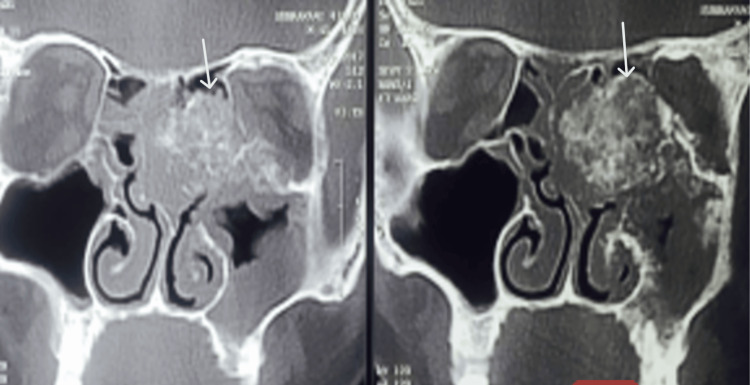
Contrast-enhanced computed tomography of the paranasal sinuses and orbit showing a heterogeneously enhancing mass arising from the left maxillary sinus with cortical destruction and superior extension into the orbital cavity. The tumor demonstrates bone erosion and soft tissue invasion involving the inferomedial orbital wall and adjacent maxillary structures.
